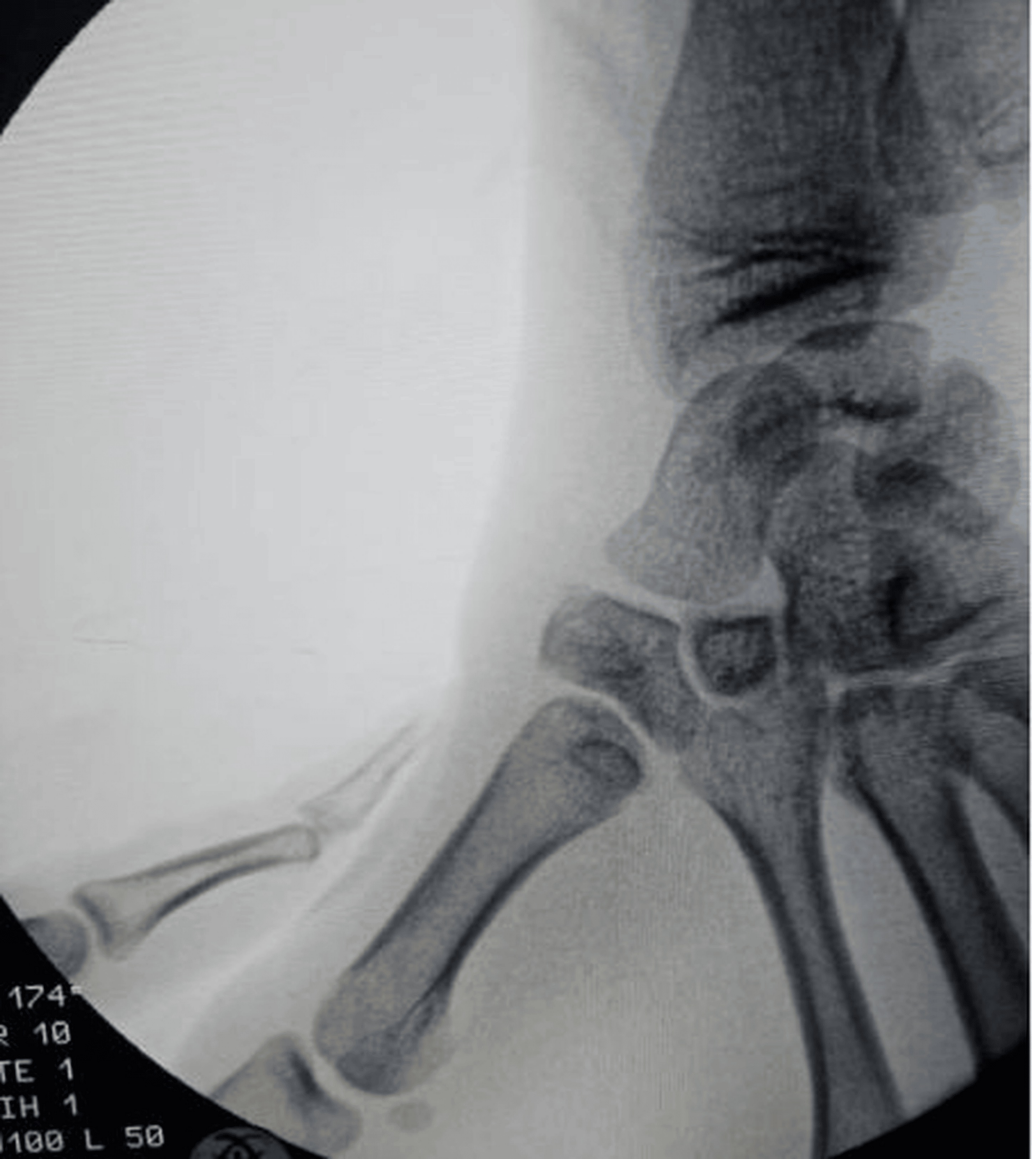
Radiographic fluoroscopy showing reduction through external manipulation.
